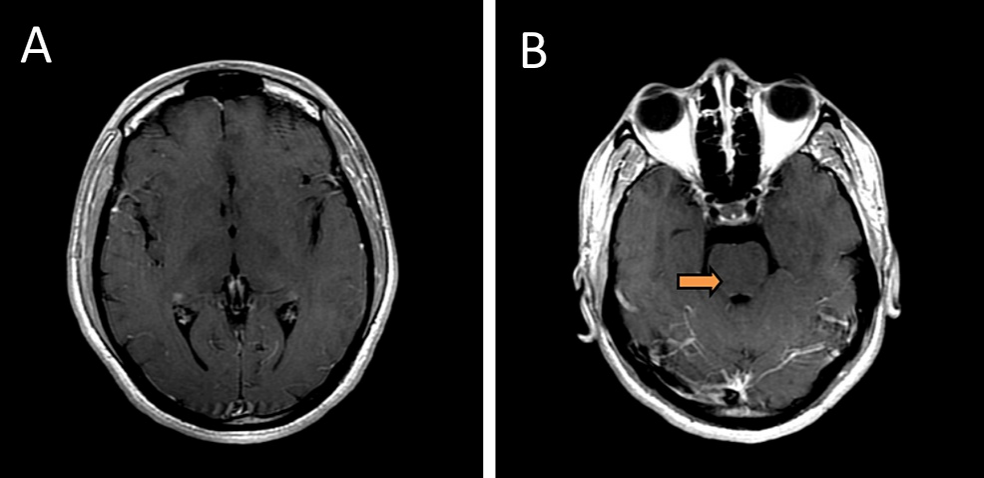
(A and B) Post-contrast T1 magnetic resonance imaging showing very subtle enhancement in the brainstem.
